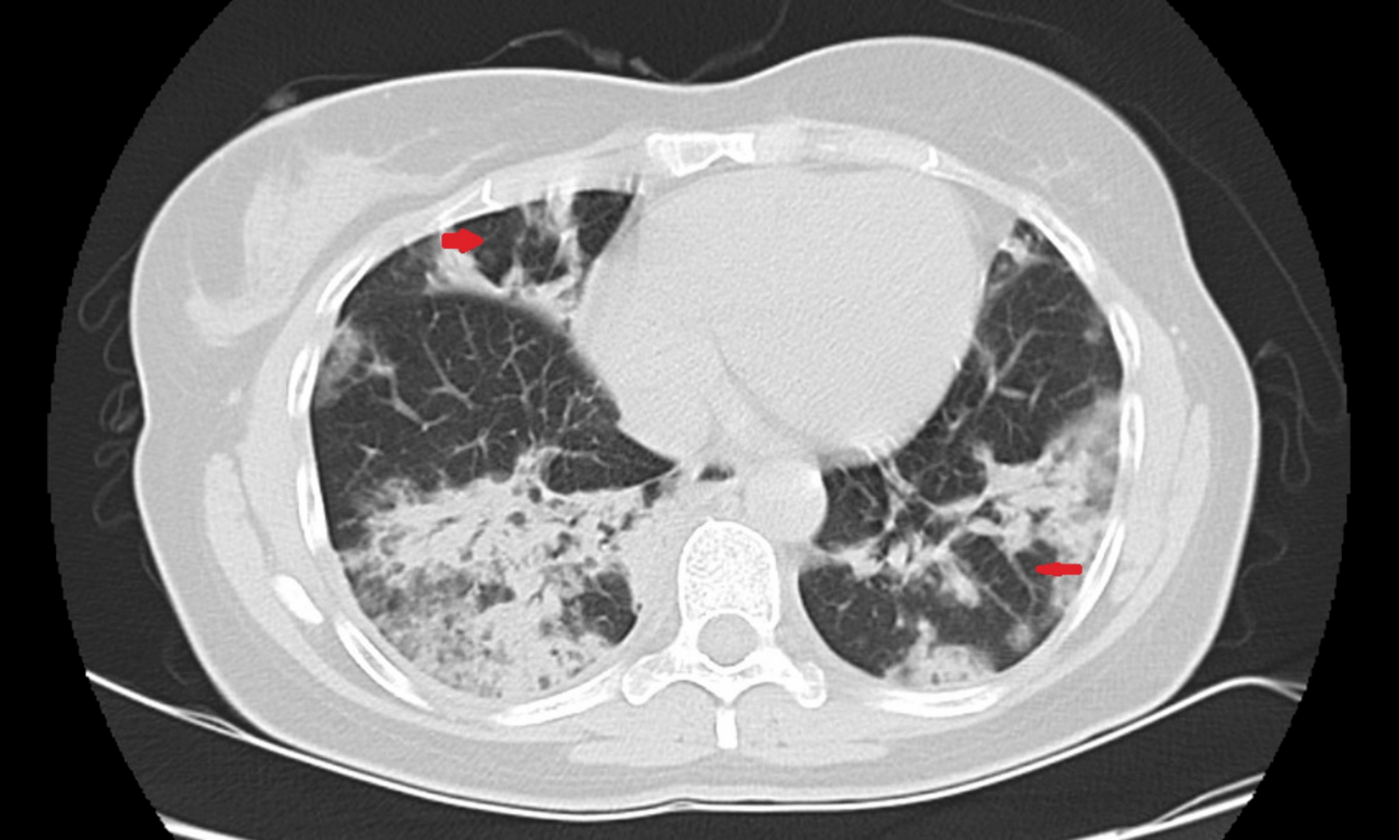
Thoracic CT-scan Red arrow indicates emphysema bubbles
